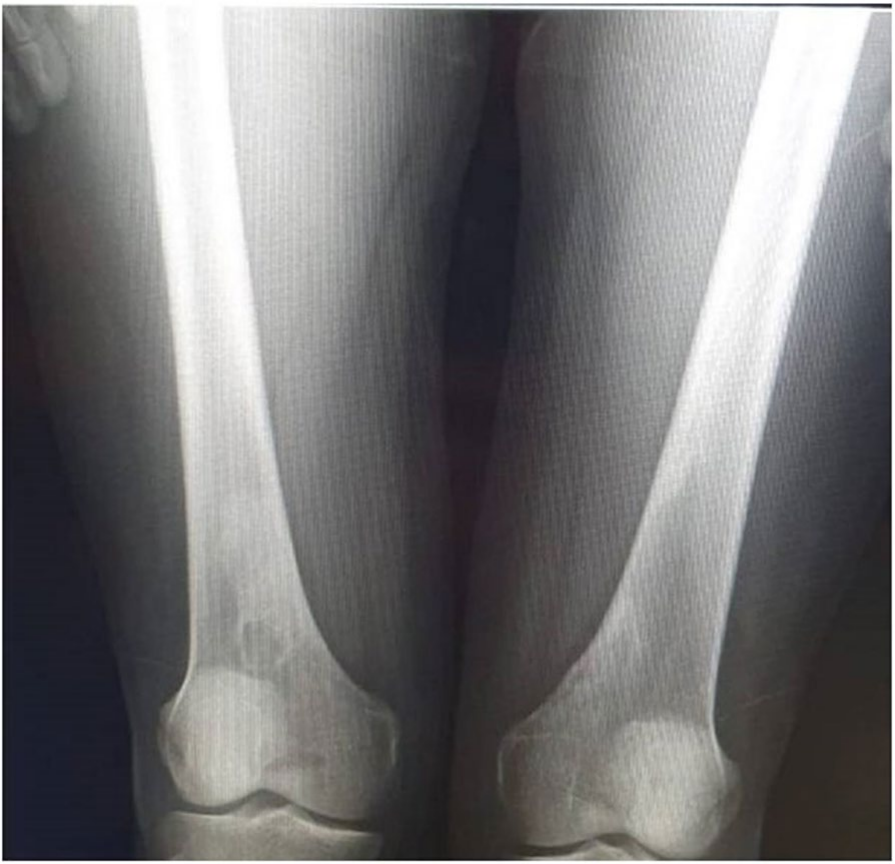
X-ray of the father shows healed NOF in the right and left distal femur
